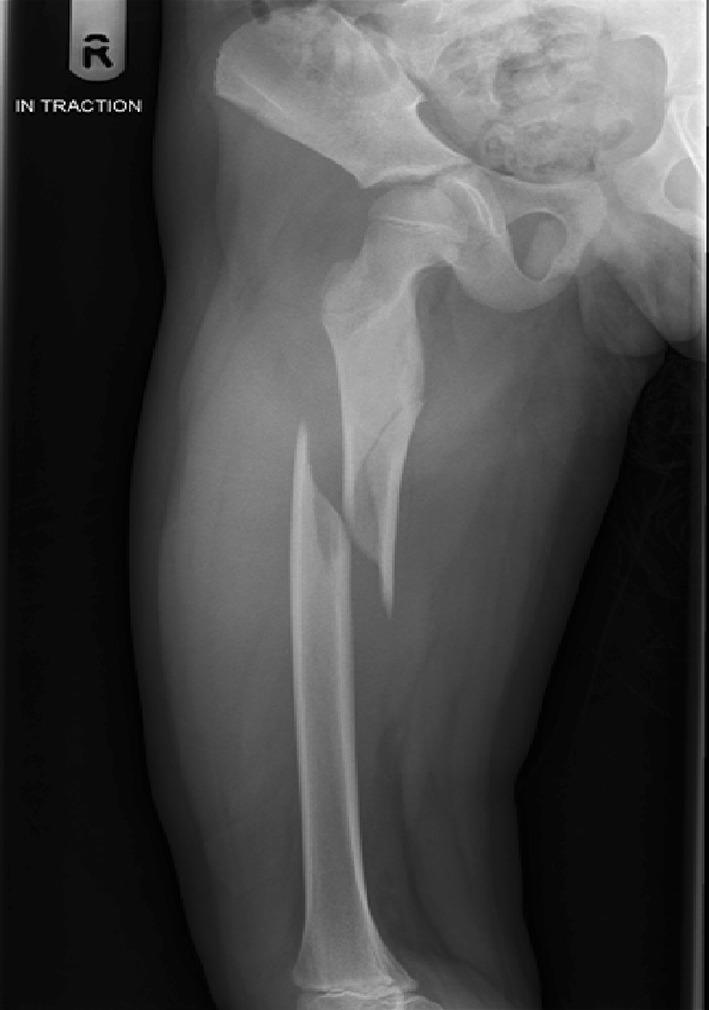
AP radiograph demonstrating a proximal third diaphyseal fracture of the femur in an 8-year-old. Note the spiral fracture with a butterfly fragment—elastic nails may be unstable here
